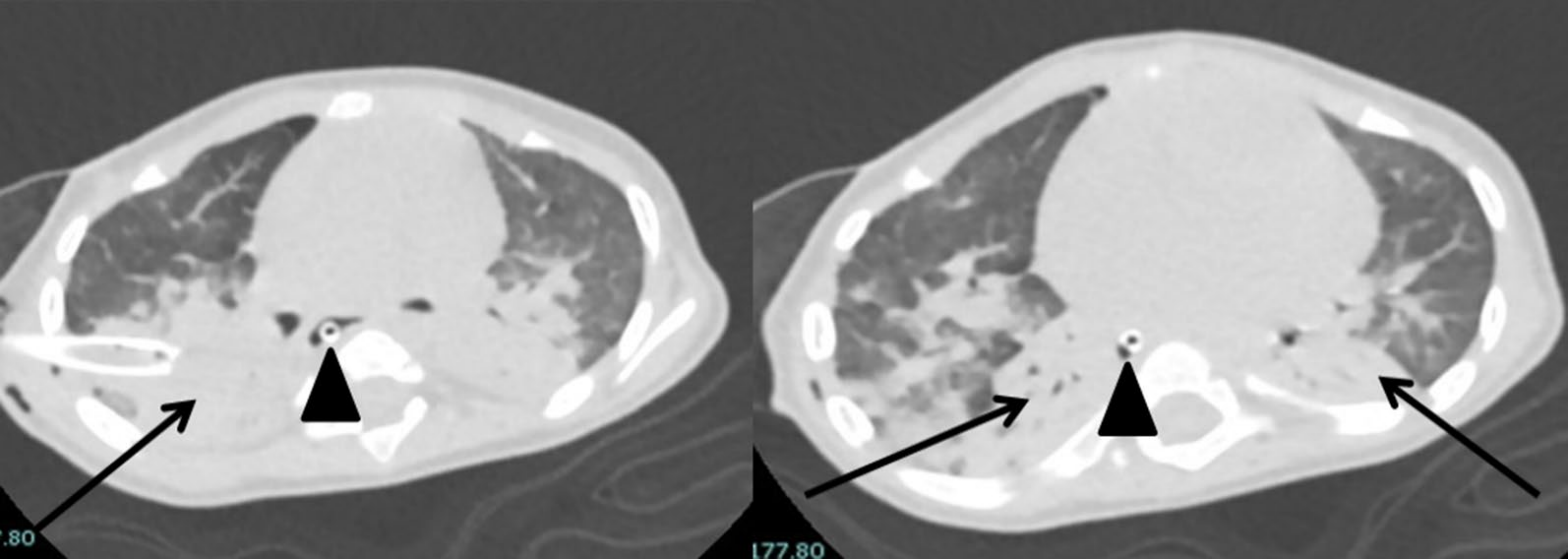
A 8-month-old female baby, known case of Down syndrome and congenital heart disease (VSD, ASD, and PDA), presented by acute dyspnea with severe respiratory manifestation. CT shows bilateral upper and lower lobar posterior consolidation and atelectasis (note chest tube is seen in the right lung due to pneumothorax (resolved)), picture atypical for COVID, and CT severity score was 15/20. *Note*: the nasogastric tube is seen slightly deviated to the right (black triangle)
